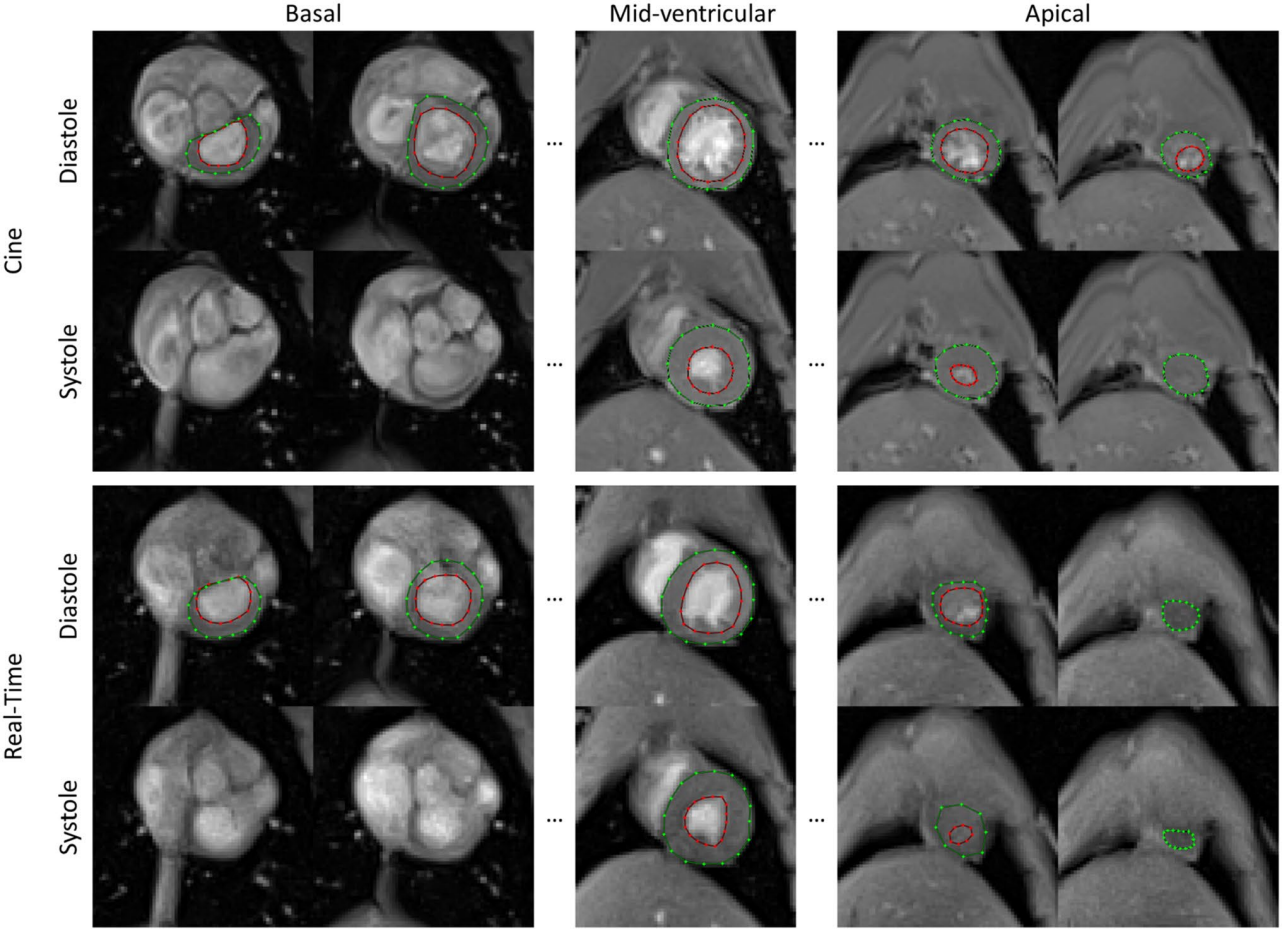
Qualitative comparison of cine MRI and RT-MRI. Compared to cine MRI (top), RT-MRI (bottom) exhibited a reduced image sharpness making the demarcation of the papillary muscles and the trabeculae challenging. Epicardial and endocardial delineations are marked in green and red, respectively. All images were acquired during expiration.
